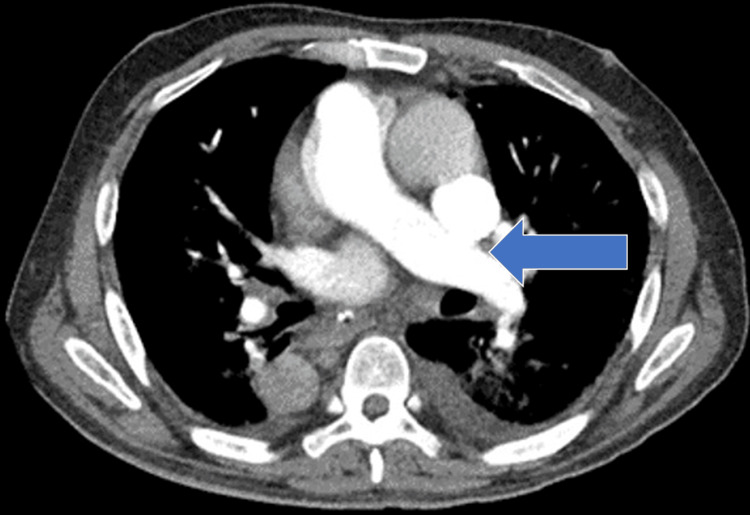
Chest computed tomography angiography (axial view) showed no filling defects which denotes absence of thrombus in the pulmonary vasculature (blue arrow)
